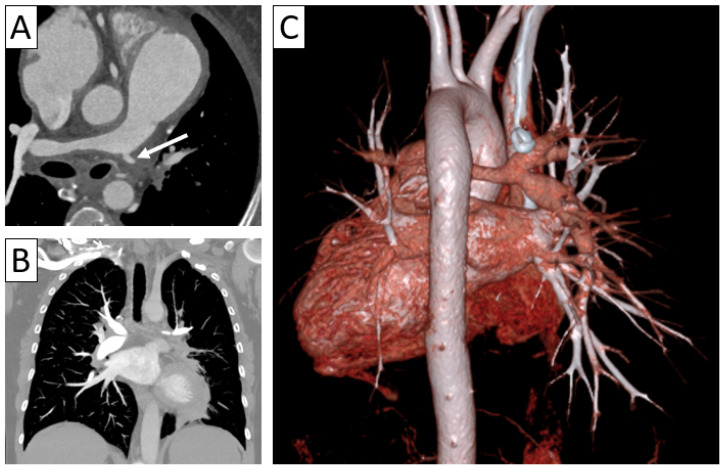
A 48-year-old female patient with Behcet’s disease presenting with oral aphthous ulcers and dyspnea. (A) Axial thoracic CTA showed diffuse thickening of the pulmonary trunk and arteries with left pulmonary artery stenosis (arrow) with extension to the lobar, segmental, and subsegmental left pulmonary arteries and subsequent vascular paucity and reduced volume of the left lung seen on coronal reformatting (B) and 3D volume rendering (C).
